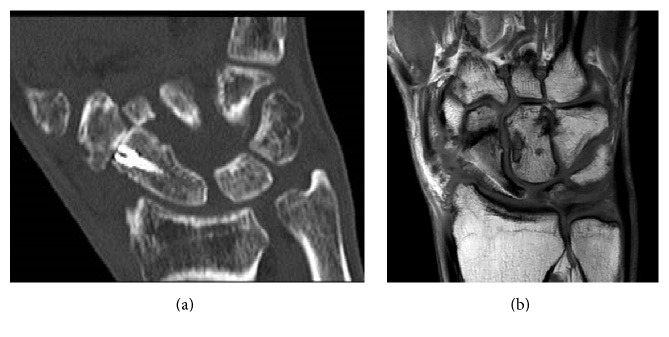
(a) CT-scan showing consolidated fracture of the scaphoid and progression of osteoarthritis in STT-joint. (b) MRI-scan showing healed fracture of the scaphoid with regular perfusion.
